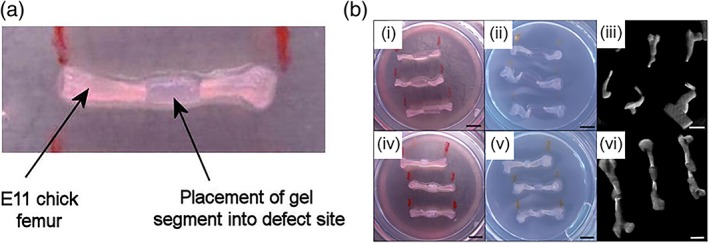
Examining the regenerative potential of solubilized bone dECM hydrogel using ex vivo model. (a) hydrogel was loaded within the 2 mm defect site created in an embryonic Day 11 chick femur. (b) Alginate hydrogel was not incorporated into the defect site after 10 days of culture (i–ii), however alginate combined with solubilized bone dECM maintained its geometry for 10 days (iv–v). Micro‐computed tomography showed similar results (iii, vi). Reprinted from Ref. 78, Copyright 2014, with permission from Elsevier
